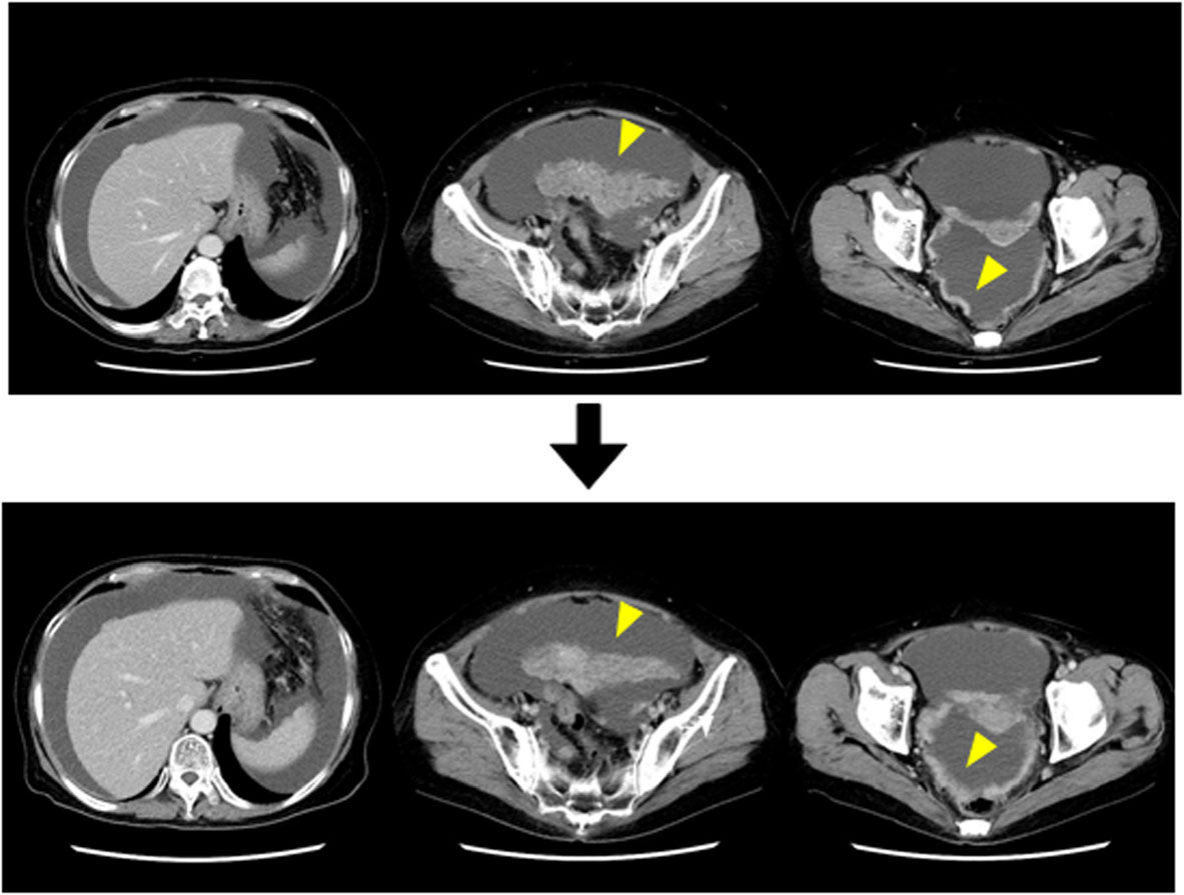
Preoperative contrast-enhanced computed tomography. Two courses of preoperative chemotherapy were administered, but the ascites and lesions in the greater omentum and peritoneum remained unchanged (arrowheads)
